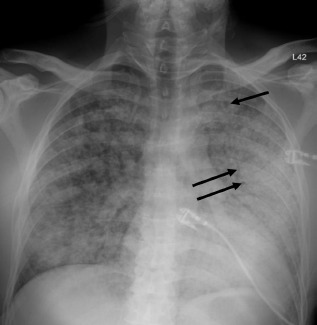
Portable chest radiograph of a 44-year-old man with hemoptysis and hypoxemic respiratory failure showing diffuse bilateral coalescent airspace opacities with air bronchograms (black arrows).
